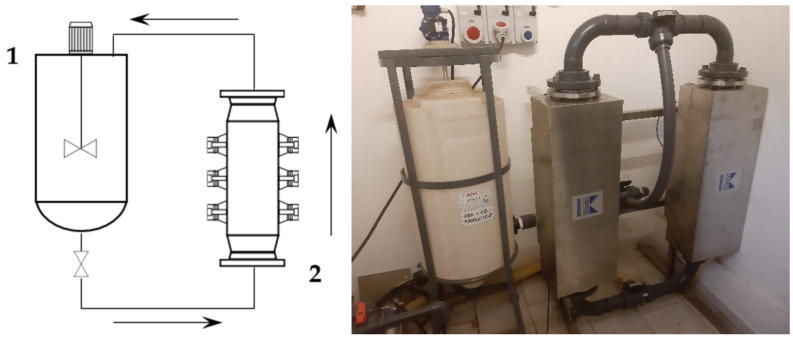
Recirculating flow system. Left: set-up scheme (1: mixed tank, 2: ultrasound (US) flow-through cell); right: facility picture.
